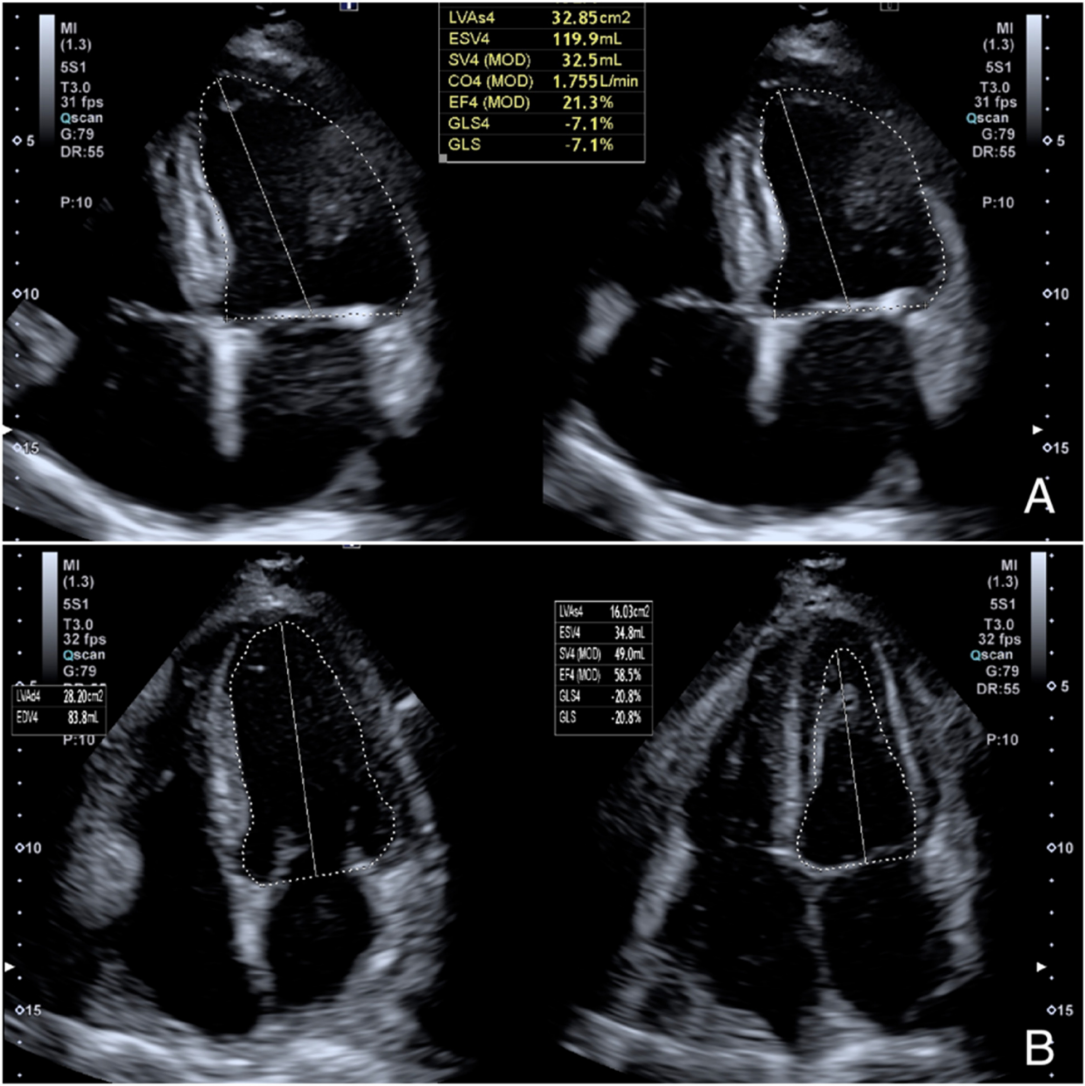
Echocardiogram (Toshiba Aplio i700 ultrasound scanner) showing in apical 4-chamber projection an LVEF of 21% calculated by Simpson's monoplane method (A); eight months after transplantation, the echocardiogram shows normal systolic function with an LVEF of 58% and a GLS of −20.8% (B) LVEF, left ventricular ejection fraction; GLS, global longitudinal strain.
